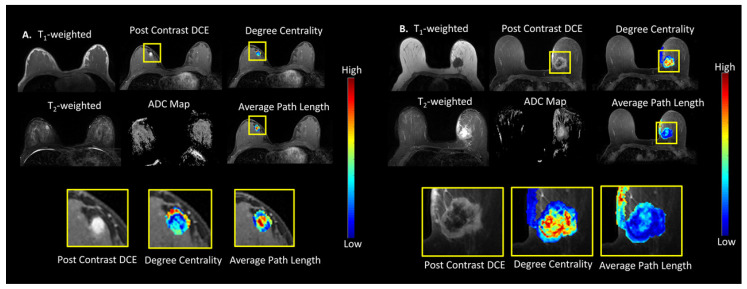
Tumor connectomics of degree centrality and average path length maps overlaid on multiparametric breast MRI. (A) An Example of a benign lesion in a 27-year-old female with focal fibroadenomatous change. The Apparent Diffusion Coefficient (ADC) Map value for this lesion was 1.54 × 10^−3^ mm^2^/s. (B) Example of an infiltrating poorly differentiated ductal carcinoma in a 56-year-old female within left breast. The ADC Map value for the lesion was 0.68 × 10^−3^ mm^2^/s.
